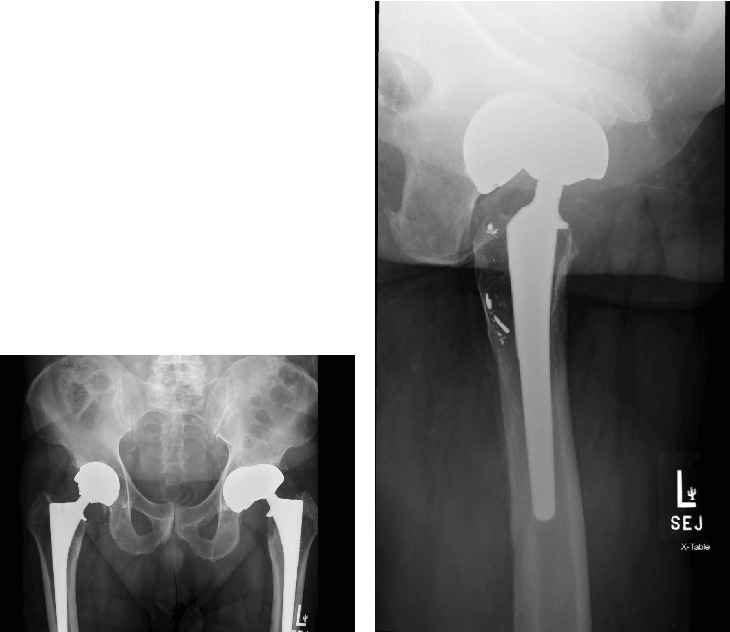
(a) Anterior–posterior (AP) radiograph demonstrating subluxation of the left THA. (b) Lateral radiograph of the left hip demonstrating anterior subluxation with metallic acetabular cup fragments situated in the area of osteolysis within the posterior aspect of the proximal femur.
